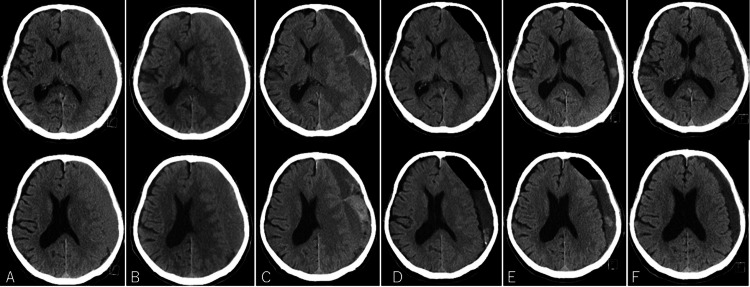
Axial non-enhanced cranial CT images. (A) At the time of the initial visit to our clinic, a CT scan of the head showed a left CSDH, but the degree of compression of the brain by the hematoma was mild. (B) When the patient noticed paralysis in the right lower leg, the cranial CT revealed an increase in the left CSDH. (C) When the patient became unconscious, the left CSDH had further increased, resulting in brain compression and deviation. (D) On the first postoperative day, cranial CT showed that the hematoma had largely been removed, with no brain compression observed. (E) Cranial CT at discharge showed no recurrence of CSDH. (F) Post-discharge cranial CT showed shrinkage of the remaining hematoma. CT, computed tomography; CSDH, chronic subdural hematoma
